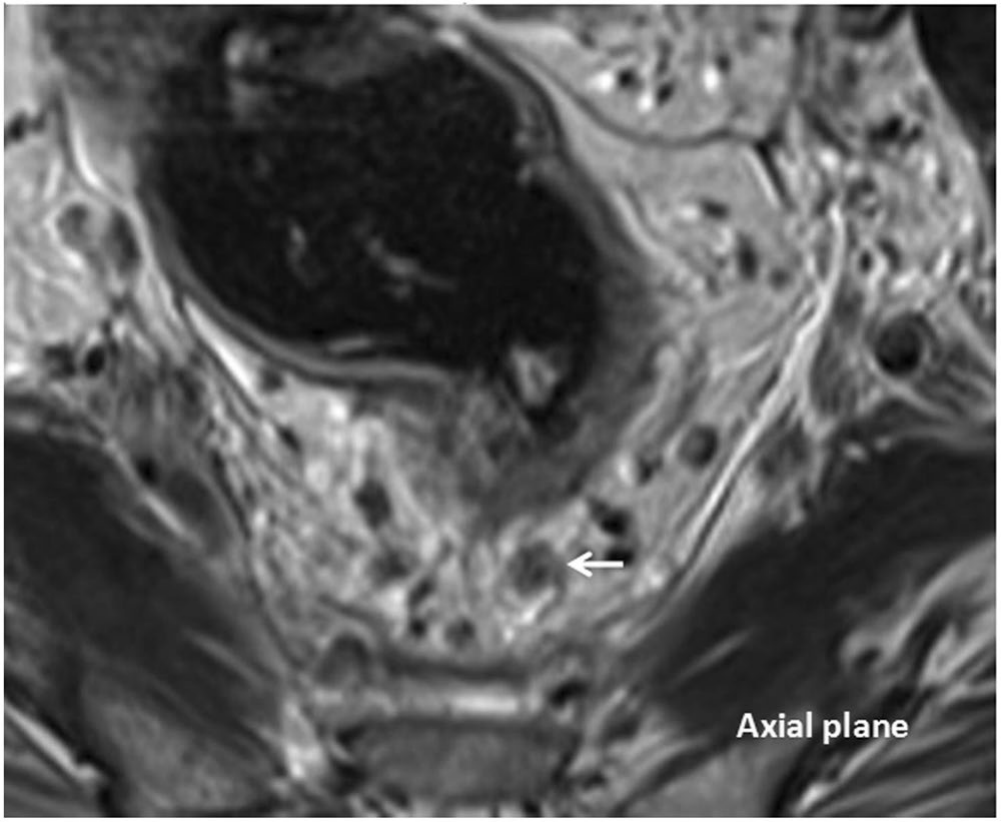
T2-weighted axial magnetic resonance images. Magnetic resonance imaging–detected positive lymph node (MR-LN): the short diameter of this lymph node is greater than 5 millimeters and the border is irregular and the signal is heterogeneous (the white arrow).
